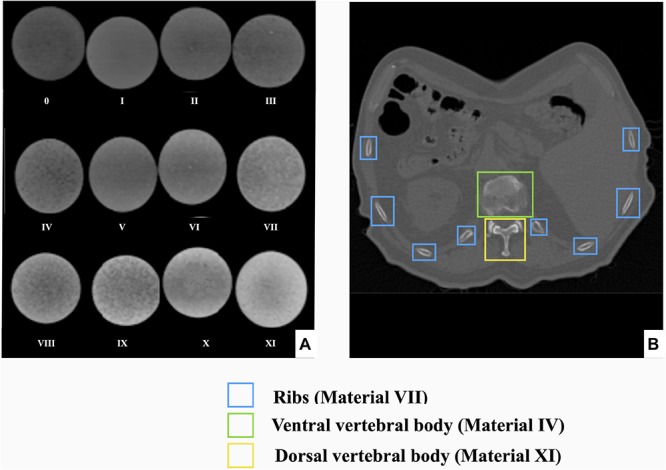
Radiodensity analysis of radiopaque amalgamate samples and their corresponding anatomic structures in human thorax. (A) CT scan of 12 different compositions of the cured radiopaque amalgamates for radiodensity analysis, (B) Axial section of CT from a patient thorax displaying that the radiodensities of the ribs, ventral and dorsal vertebral bodies were replicated by radiopaque amalgamates VII, IV, and XI, respectively.
